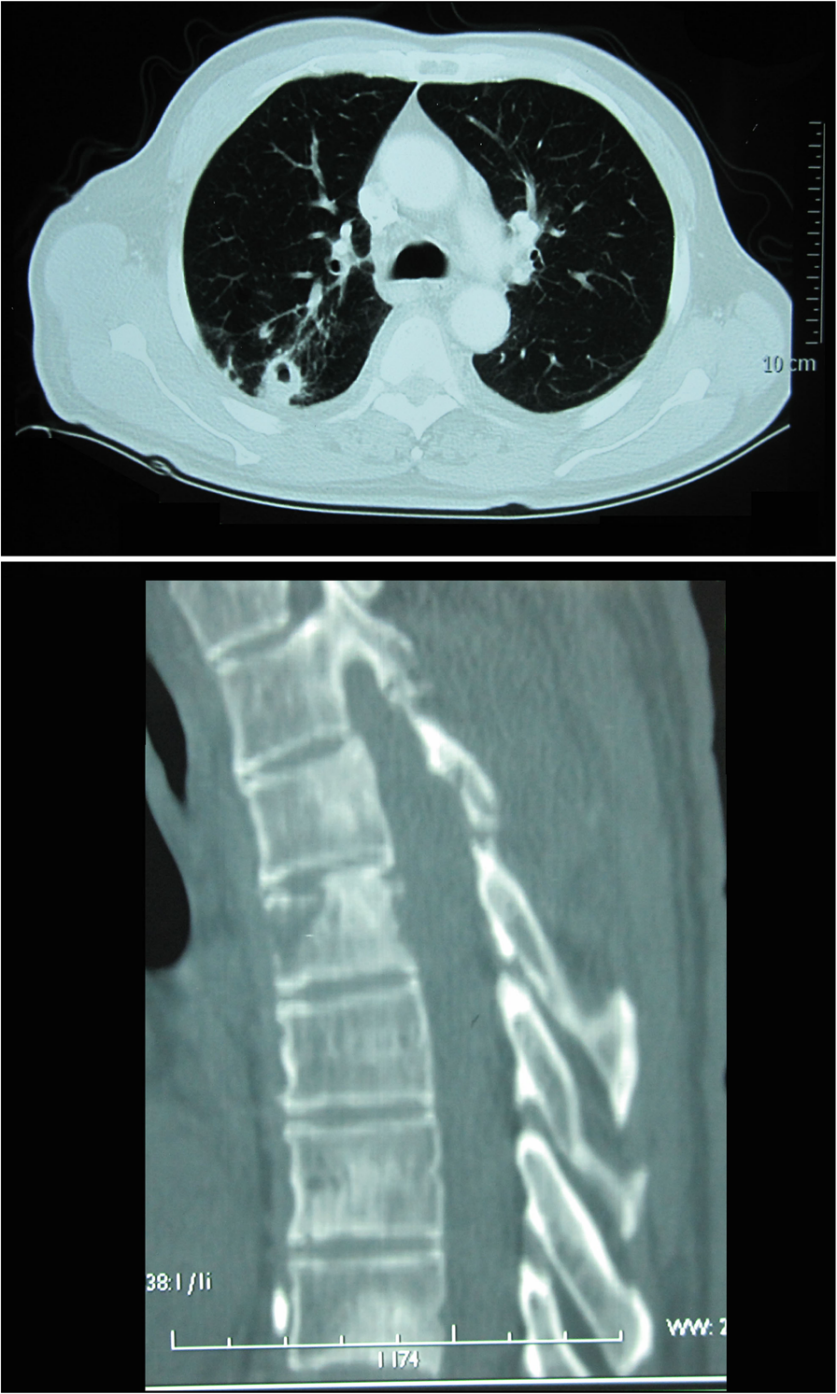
CT scan revealed progressing infection: Chest CT scan revealed the unresolved pulmonary abscess (*above*) compared with 2 months before (Fig. 2). Moreover, there were additional multiple destructions of vertebral bodies, especially T5 in the figure below
